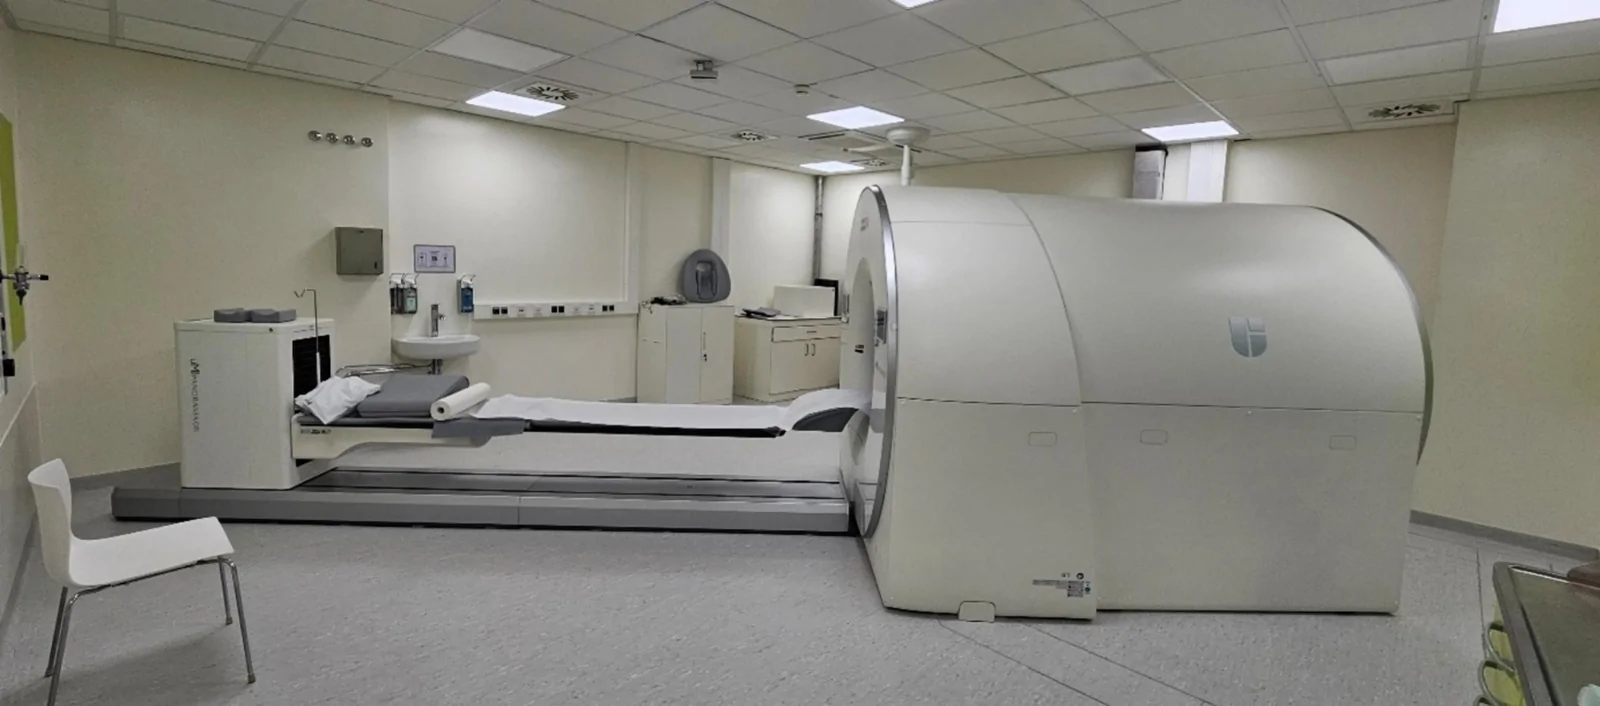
Photograph of United Imaging Healthcare - Panorama GS LAFOV PET/CT installed at Hannover Medical School
